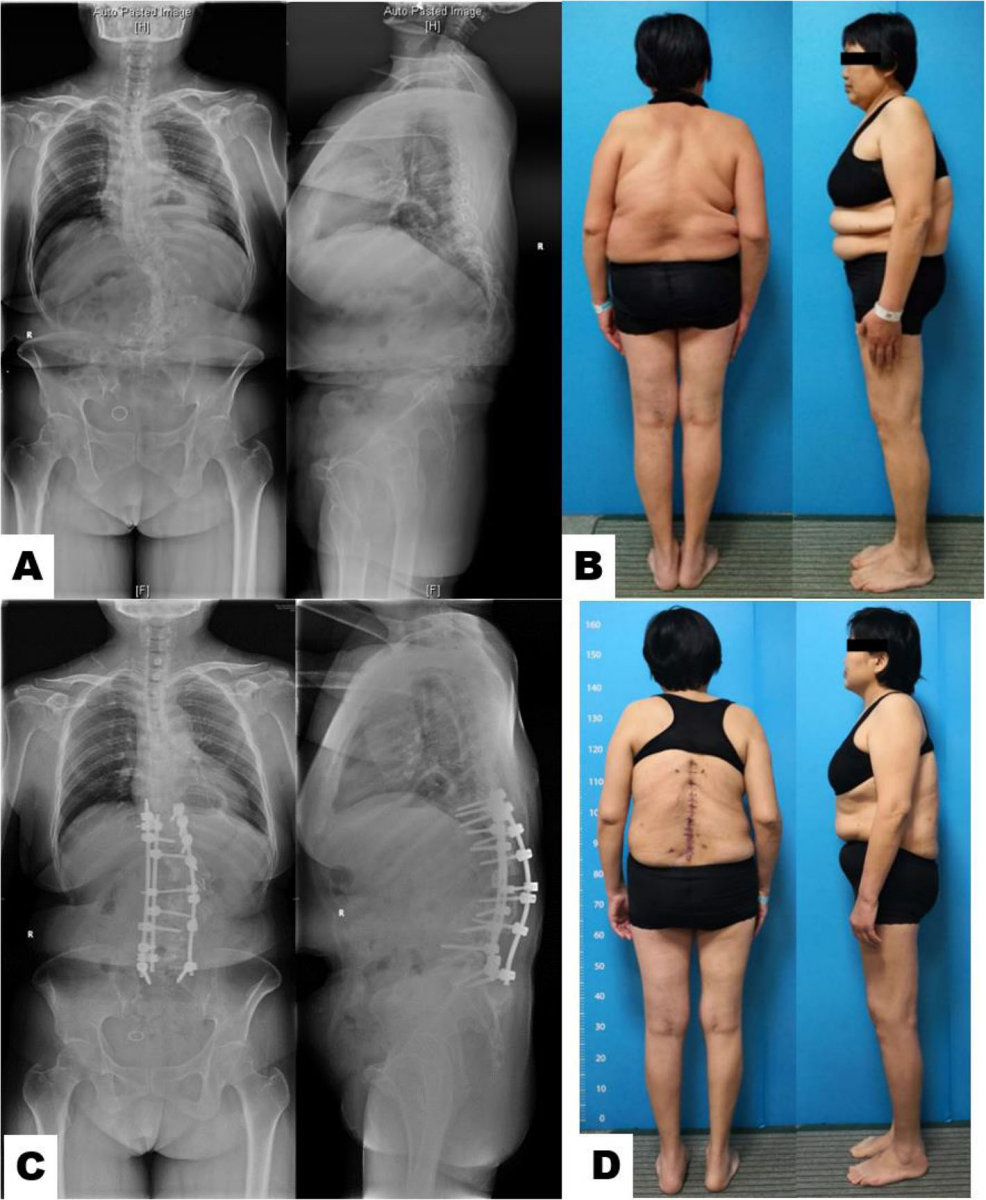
A 52-year-old woman of complex and rigid congenital kyphoscoliosis (L1/2 failure of segmentation) with obesity. She underwent spinal fusion from T9 to S1 and L1/L2 VCR. a Patients’ preoperative standing spine radiograph demonstrated the main curve of 88.3° and 92° thoracolumbar kyphosis with grade IV AVR. b Preoperative appearance photograph. c Postoperative standing spine radiograph showed the main curve was improved to 48°, with a correction rate of 45.6%. d Patients’ postoperative appearance photograph
